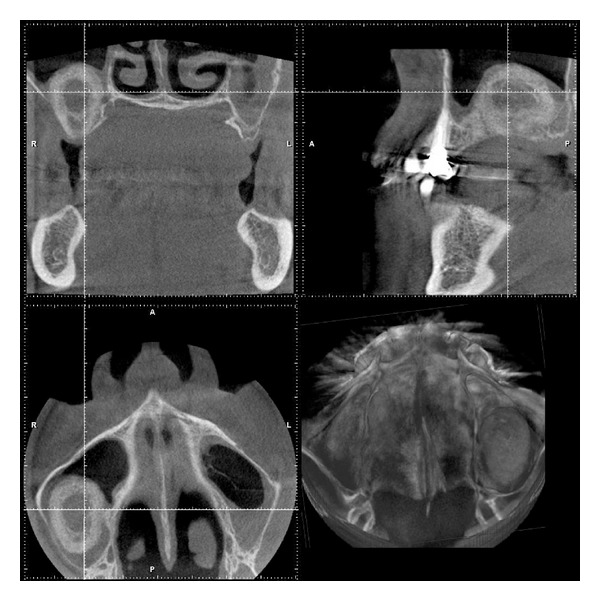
Case 2: CBCT scan 4 months following tHUCSL-Intralift. The even circular centripetal calcification process can be observed.
